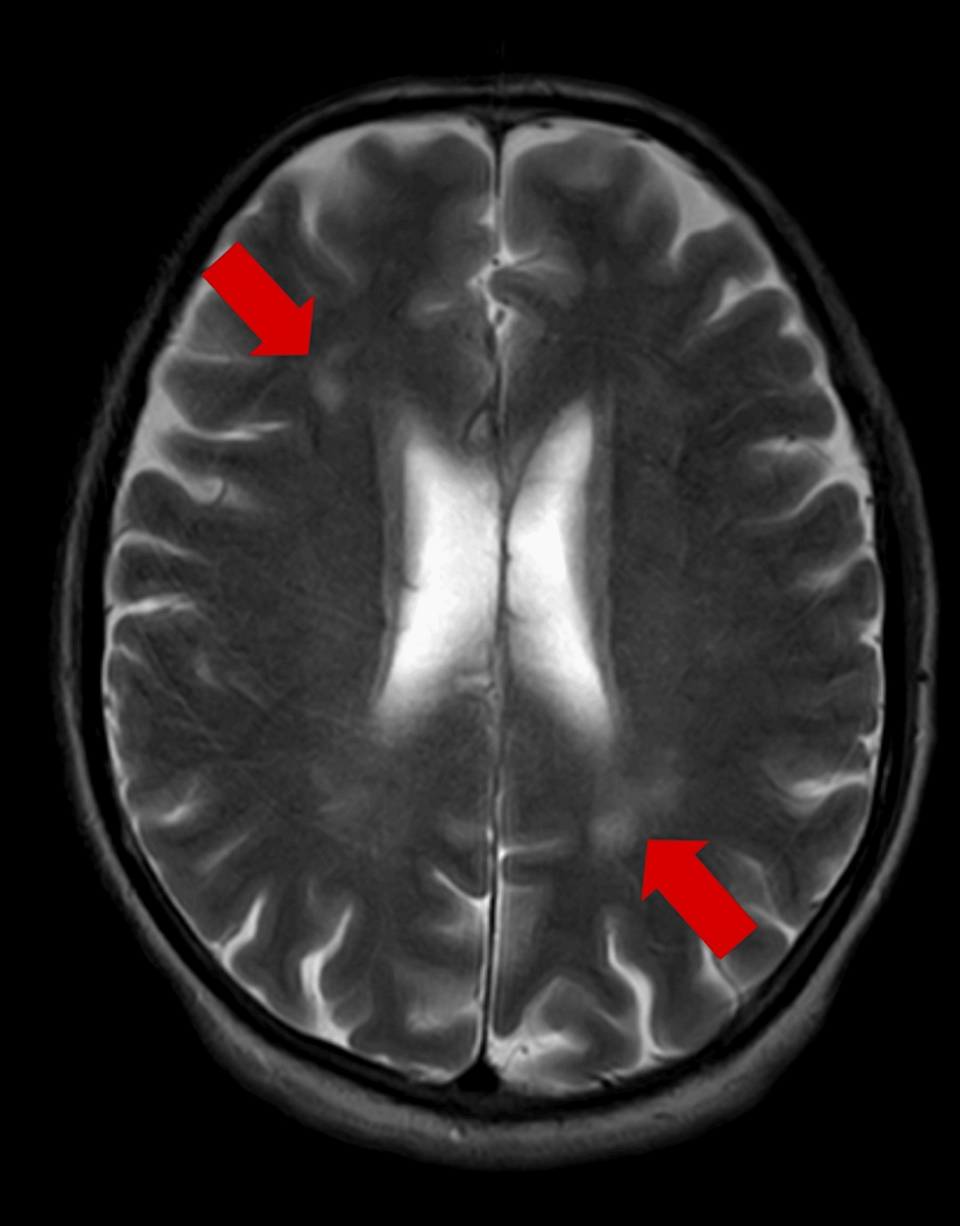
Noncontrast MRI brain image: T2 axial section showing hyperintensity in the bilateral subcortical and periventricular deep white matter The patient's brain volume is good and no cortical atrophy is observed in terms of dementia.
